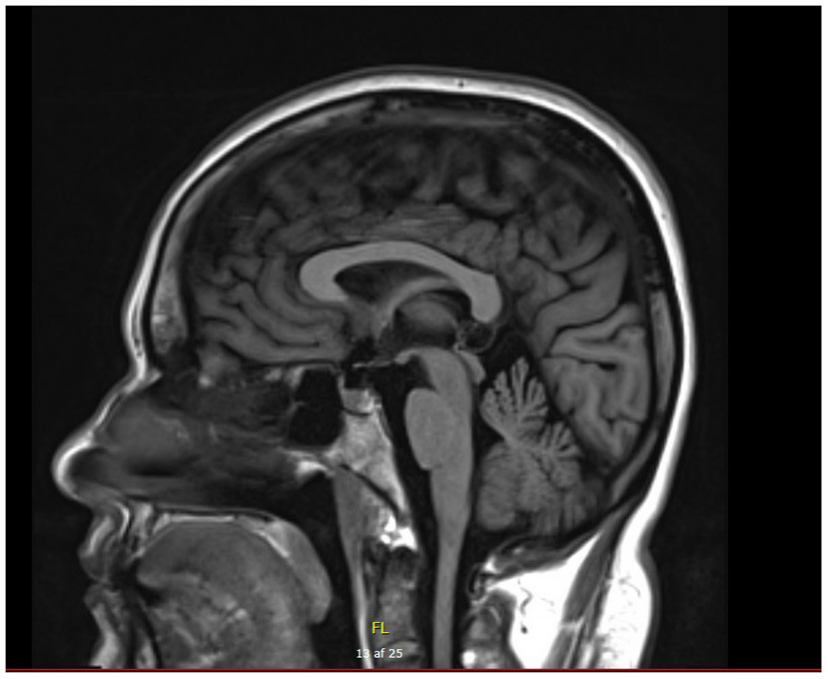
Cerebral MRI of patient B with cerebellar atrophy involving vermis.
